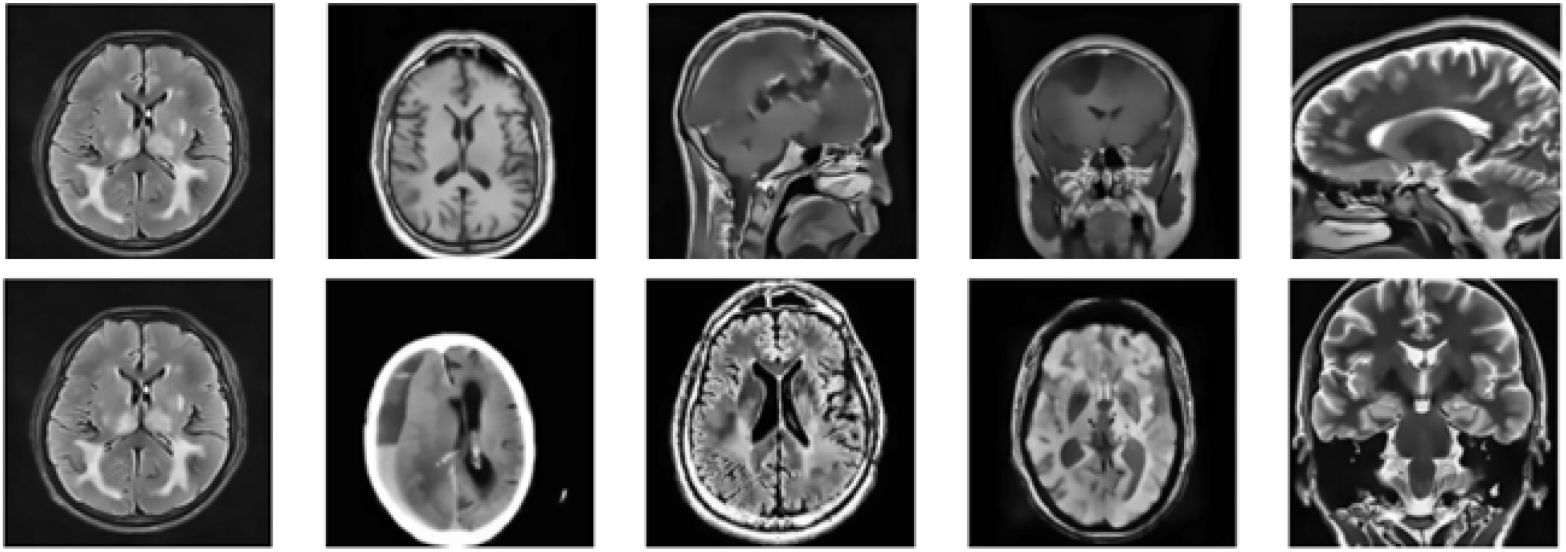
A few MRI images showing both benign and malignant cases.
